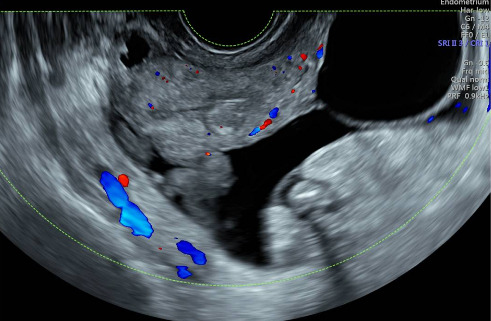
Transvaginal pelvic ultrasound showing a complex tumor dependent on the left ovary, with plenty of free peritoneal fluid in the pelvic cavity.
